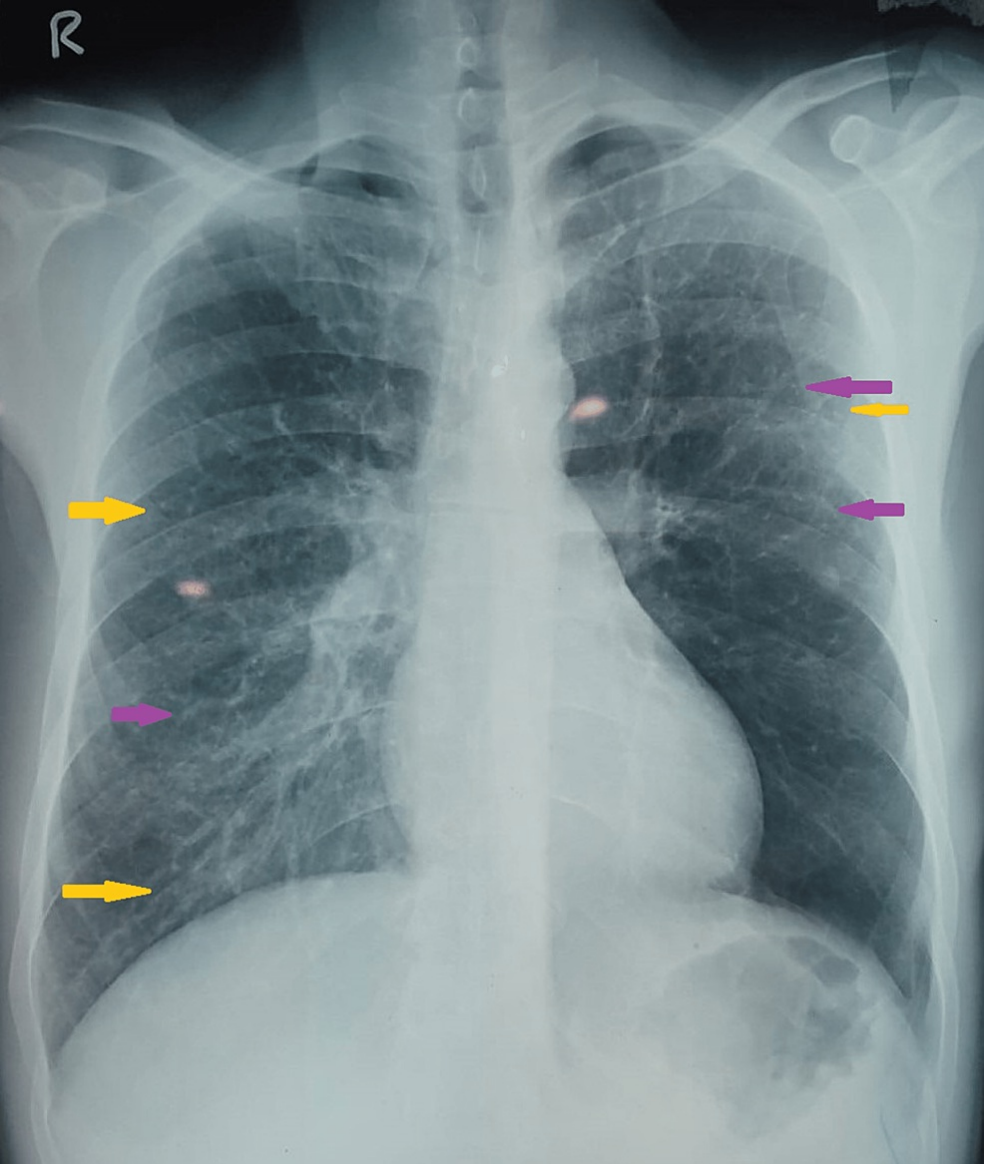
Chest radiograph showing reticulonodular opacities involving all zones of both lung fields Purple arrows indicate reticular opacities. Yellow arrows indicate nodular opacities.
